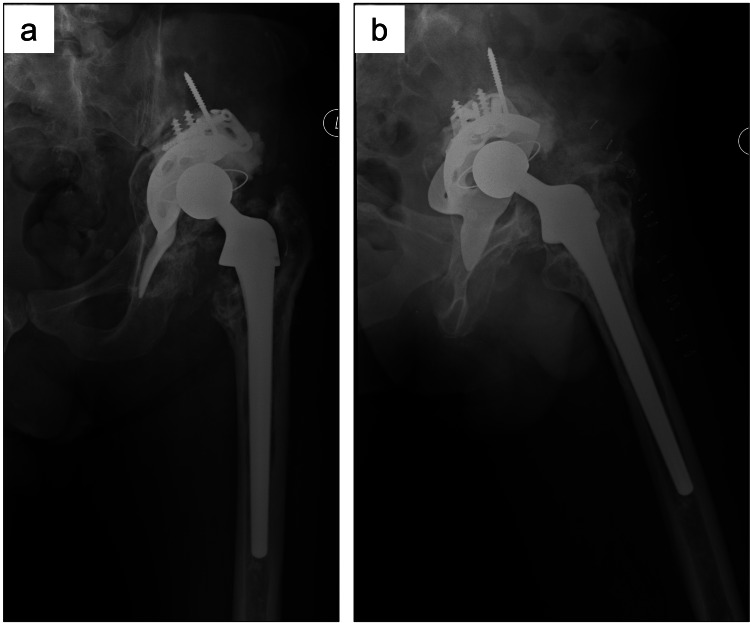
Postoperative hip radiographs a, b: Obtained in 2009 after removal of the Lord loose cup and implantation of a Burch-Schneider (Zimmer Biomet, IN, USA) cage with a cemented polyethylene cup. The massive acetabular bone defect was reconstructed using impaction bone allografting, and the femoral stem was retained.
